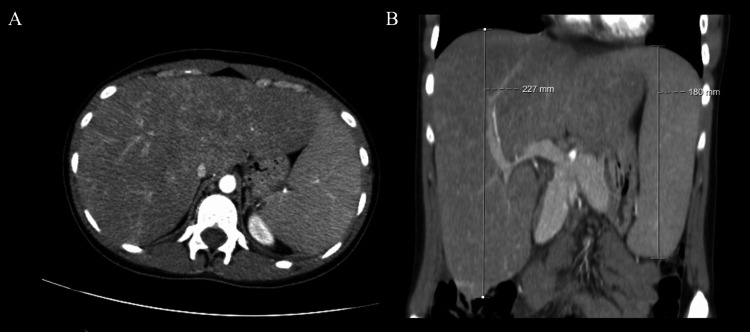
Hepatosplenomegaly on abdominal CT scan. A: axial plane; B: coronal plane
